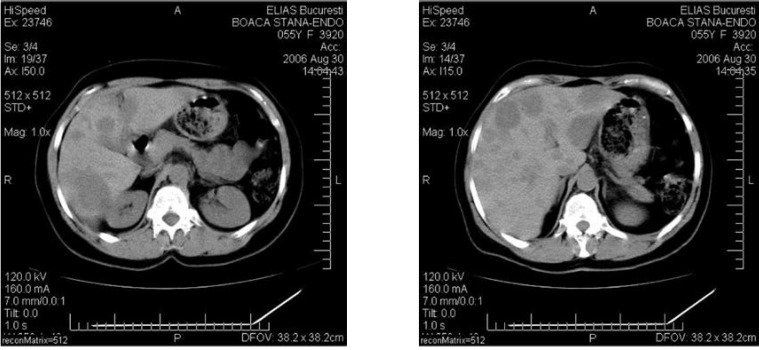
Abdominal CT revealed the progression of pancreatic tumor at 2,5 cm and the increase number and dimension of liver metastasis ( august 2006)
